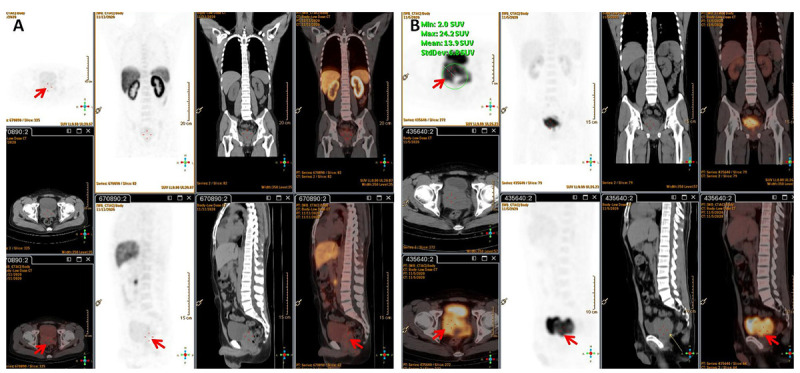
(A) 18F-PSMA PET/CT showed no trace PSMA uptake in the lesion (red arrow). (B) 18F-FDG PET/CT revealed foci with increased glucose metabolism in the prostate and bilateral seminal vesicles. Multiple enlarged lymph nodes with varying degrees of increased glucose metabolism were observed in the hilum, retroperitoneum, and pelvic cavity.
